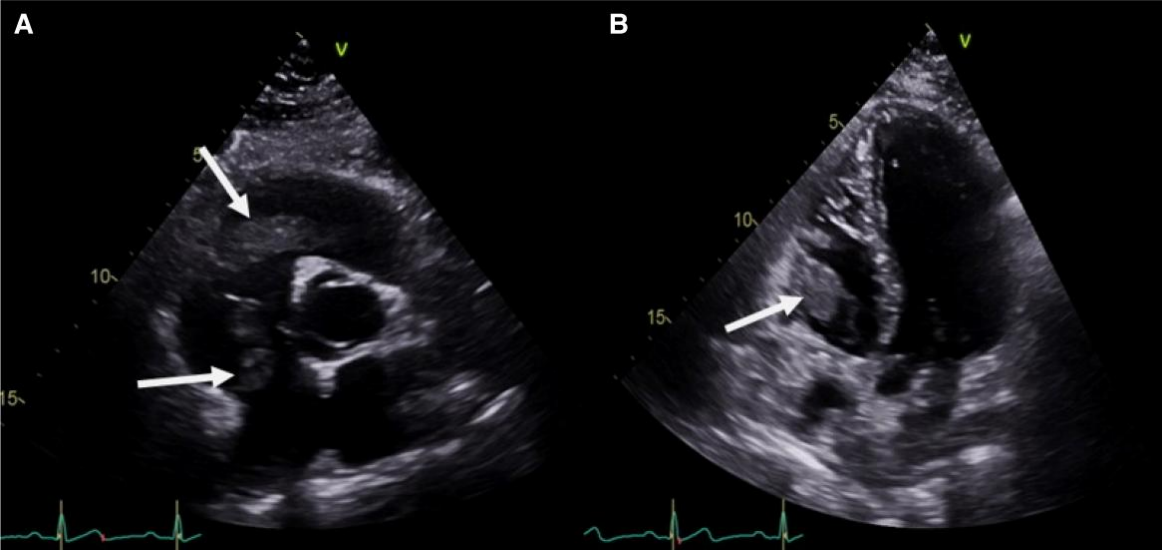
Two-dimensional transthoracic echocardiography reveals multiple thrombi in the right cavities (*A*)—parasternal short axis view showing one thrombus in the right atrium close to the septal leaflet of the tricuspid valve and one thrombus in the right ventricle attached to the lateral region of the tricuspid annulus (arrow)—(*B*) apical four-chamber view showing the thrombus at the level of the free wall of the right ventricle (arrow).
